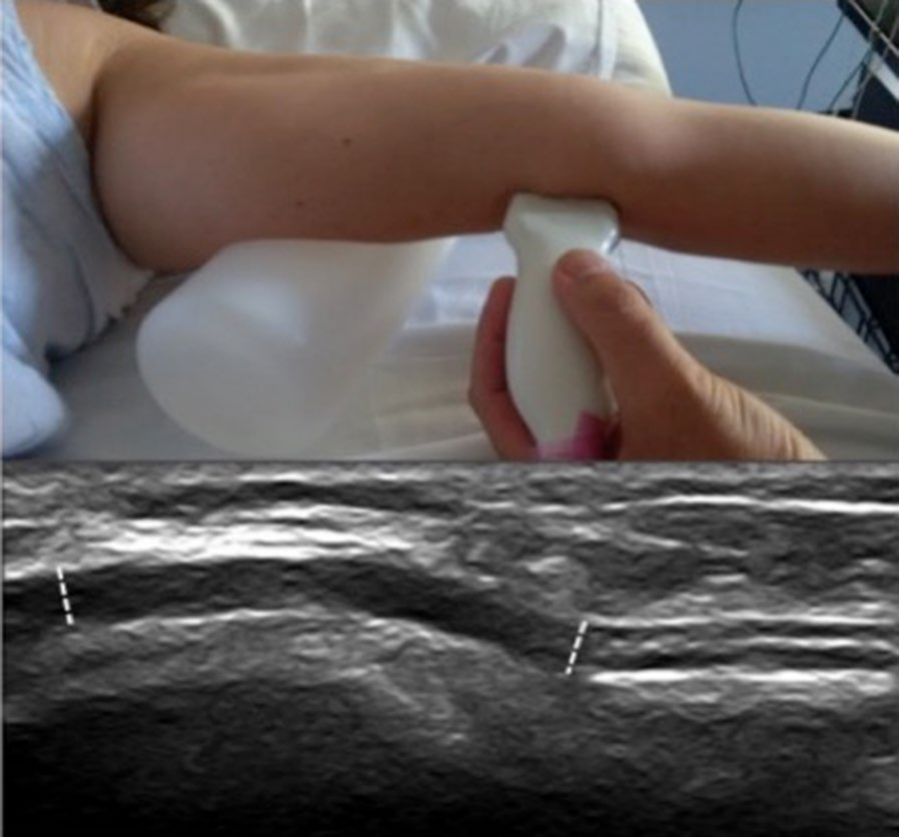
Top: position of the arm for the longitudinal ultrasound examination. Bottom: normal ultrasound of the UN at the elbow in the longitudinal evaluation; white lines indicate ASD, distal (right) and proximal (left)
